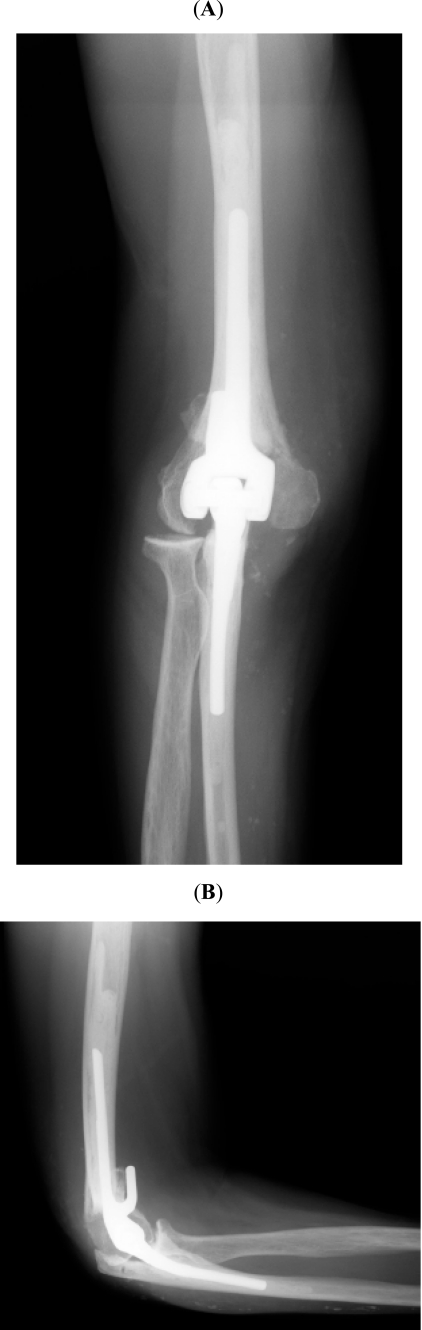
Anteroposterior (A) and lateral (B) radiographs after elbow arthroplasty for rheumatoid arthritis.
